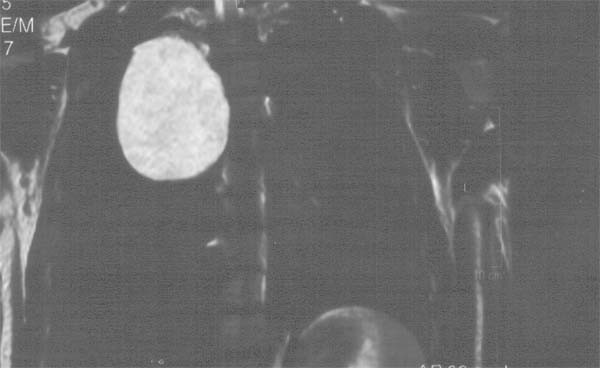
T2-W MRI scan set the diagnosis of the bronchogenic cyst and the vertebral dislodgment in the saggital section.
